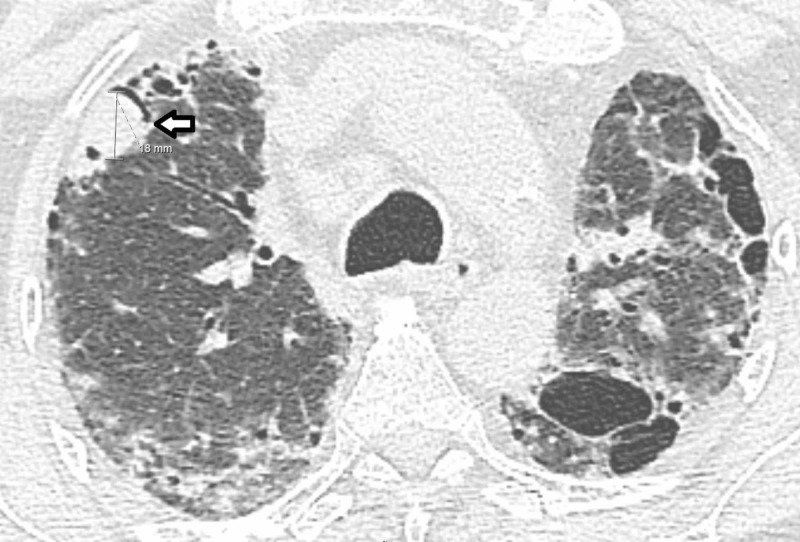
CT scan of chest without contrast demonstrating a mycetoma (left arrow) along with diffuse fibrotic changes.
